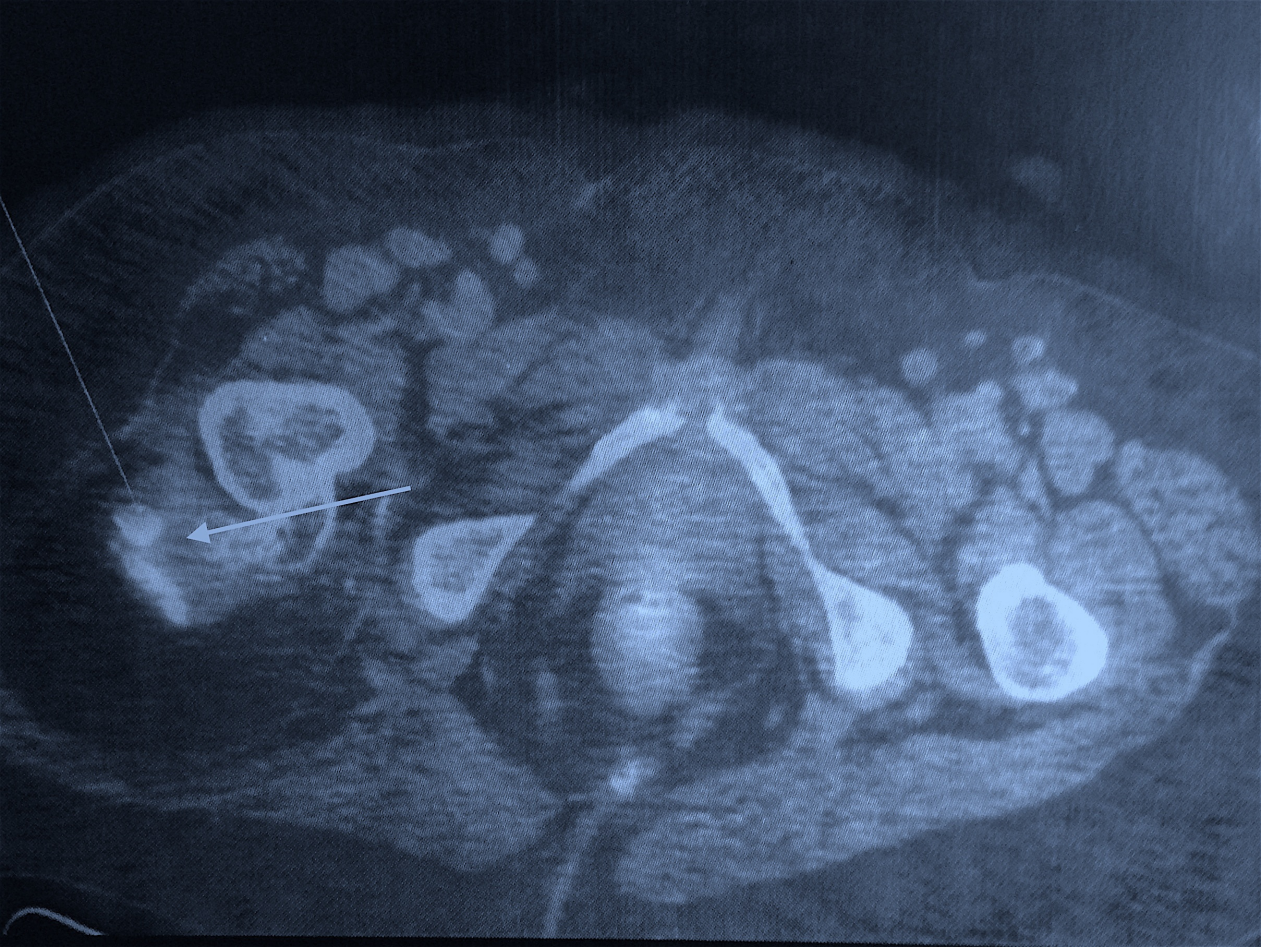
Fluoro-deoxy-glucose (FDG) bone scan showing a metabolically active lesion arising from the posterior aspect of the greater trochanter
